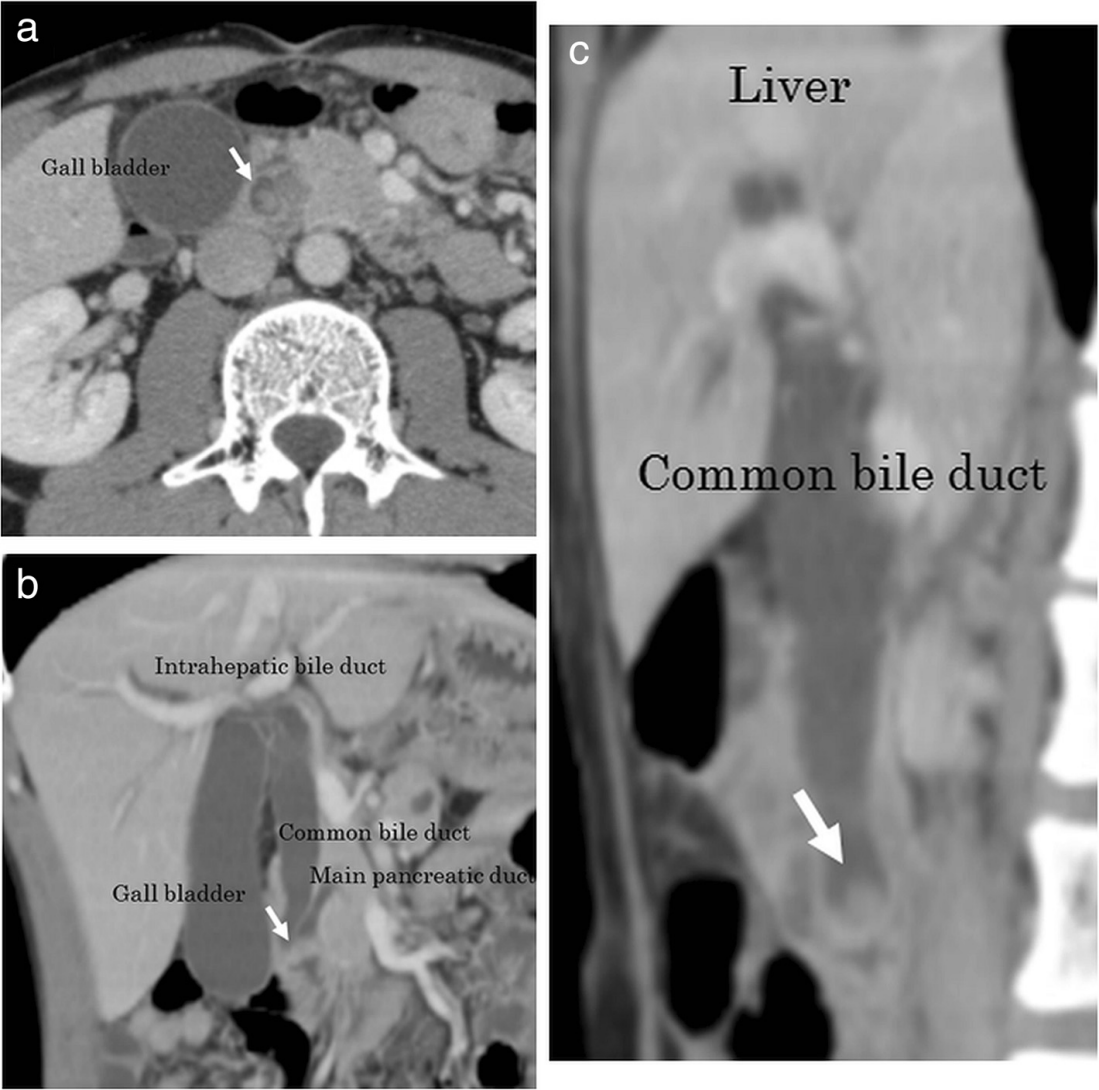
Enhanced CT image showed a hypervascular tumor, 10 mm in diameter, at the papilla of Vater (*arrow*) (a) and dilation of the common and intrahepatic bile ducts (*arrow*) (b, c)
